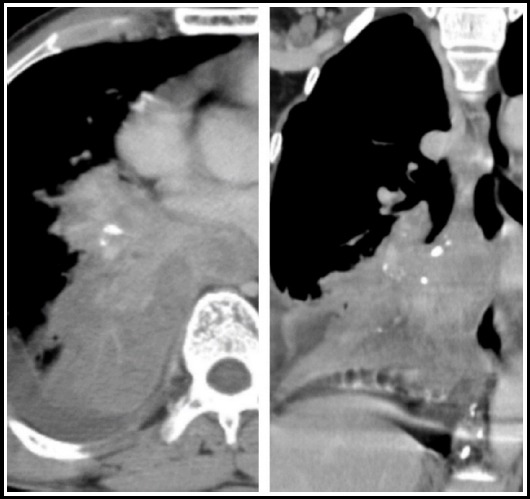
CT scan chest in mediastinal window settings shows an abnormally enhancing, spiculated infraright hilar mass lesion with specks of calcification with adjacent collapse. This patient received ATT 5 years back and now has biopsy proven bronchogenic carcinoma.
